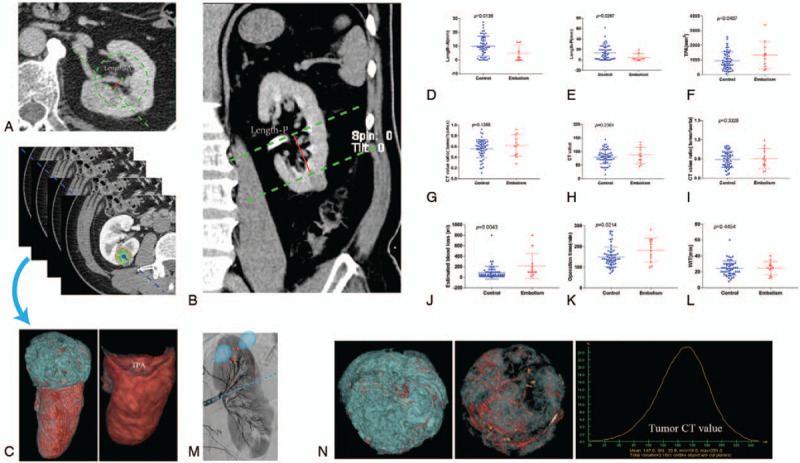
Measurement method of renal tumor-parenchyma contact area, length-A and length-P (A-C). Differences in imaging and surgical factors between embolism patients and controls (D-L). As Length-A and Length-P decreased, the order of the involved arteries increased. The red arrow shows the involved arteries (M) and measurements of the mean tumor CT value (N).
